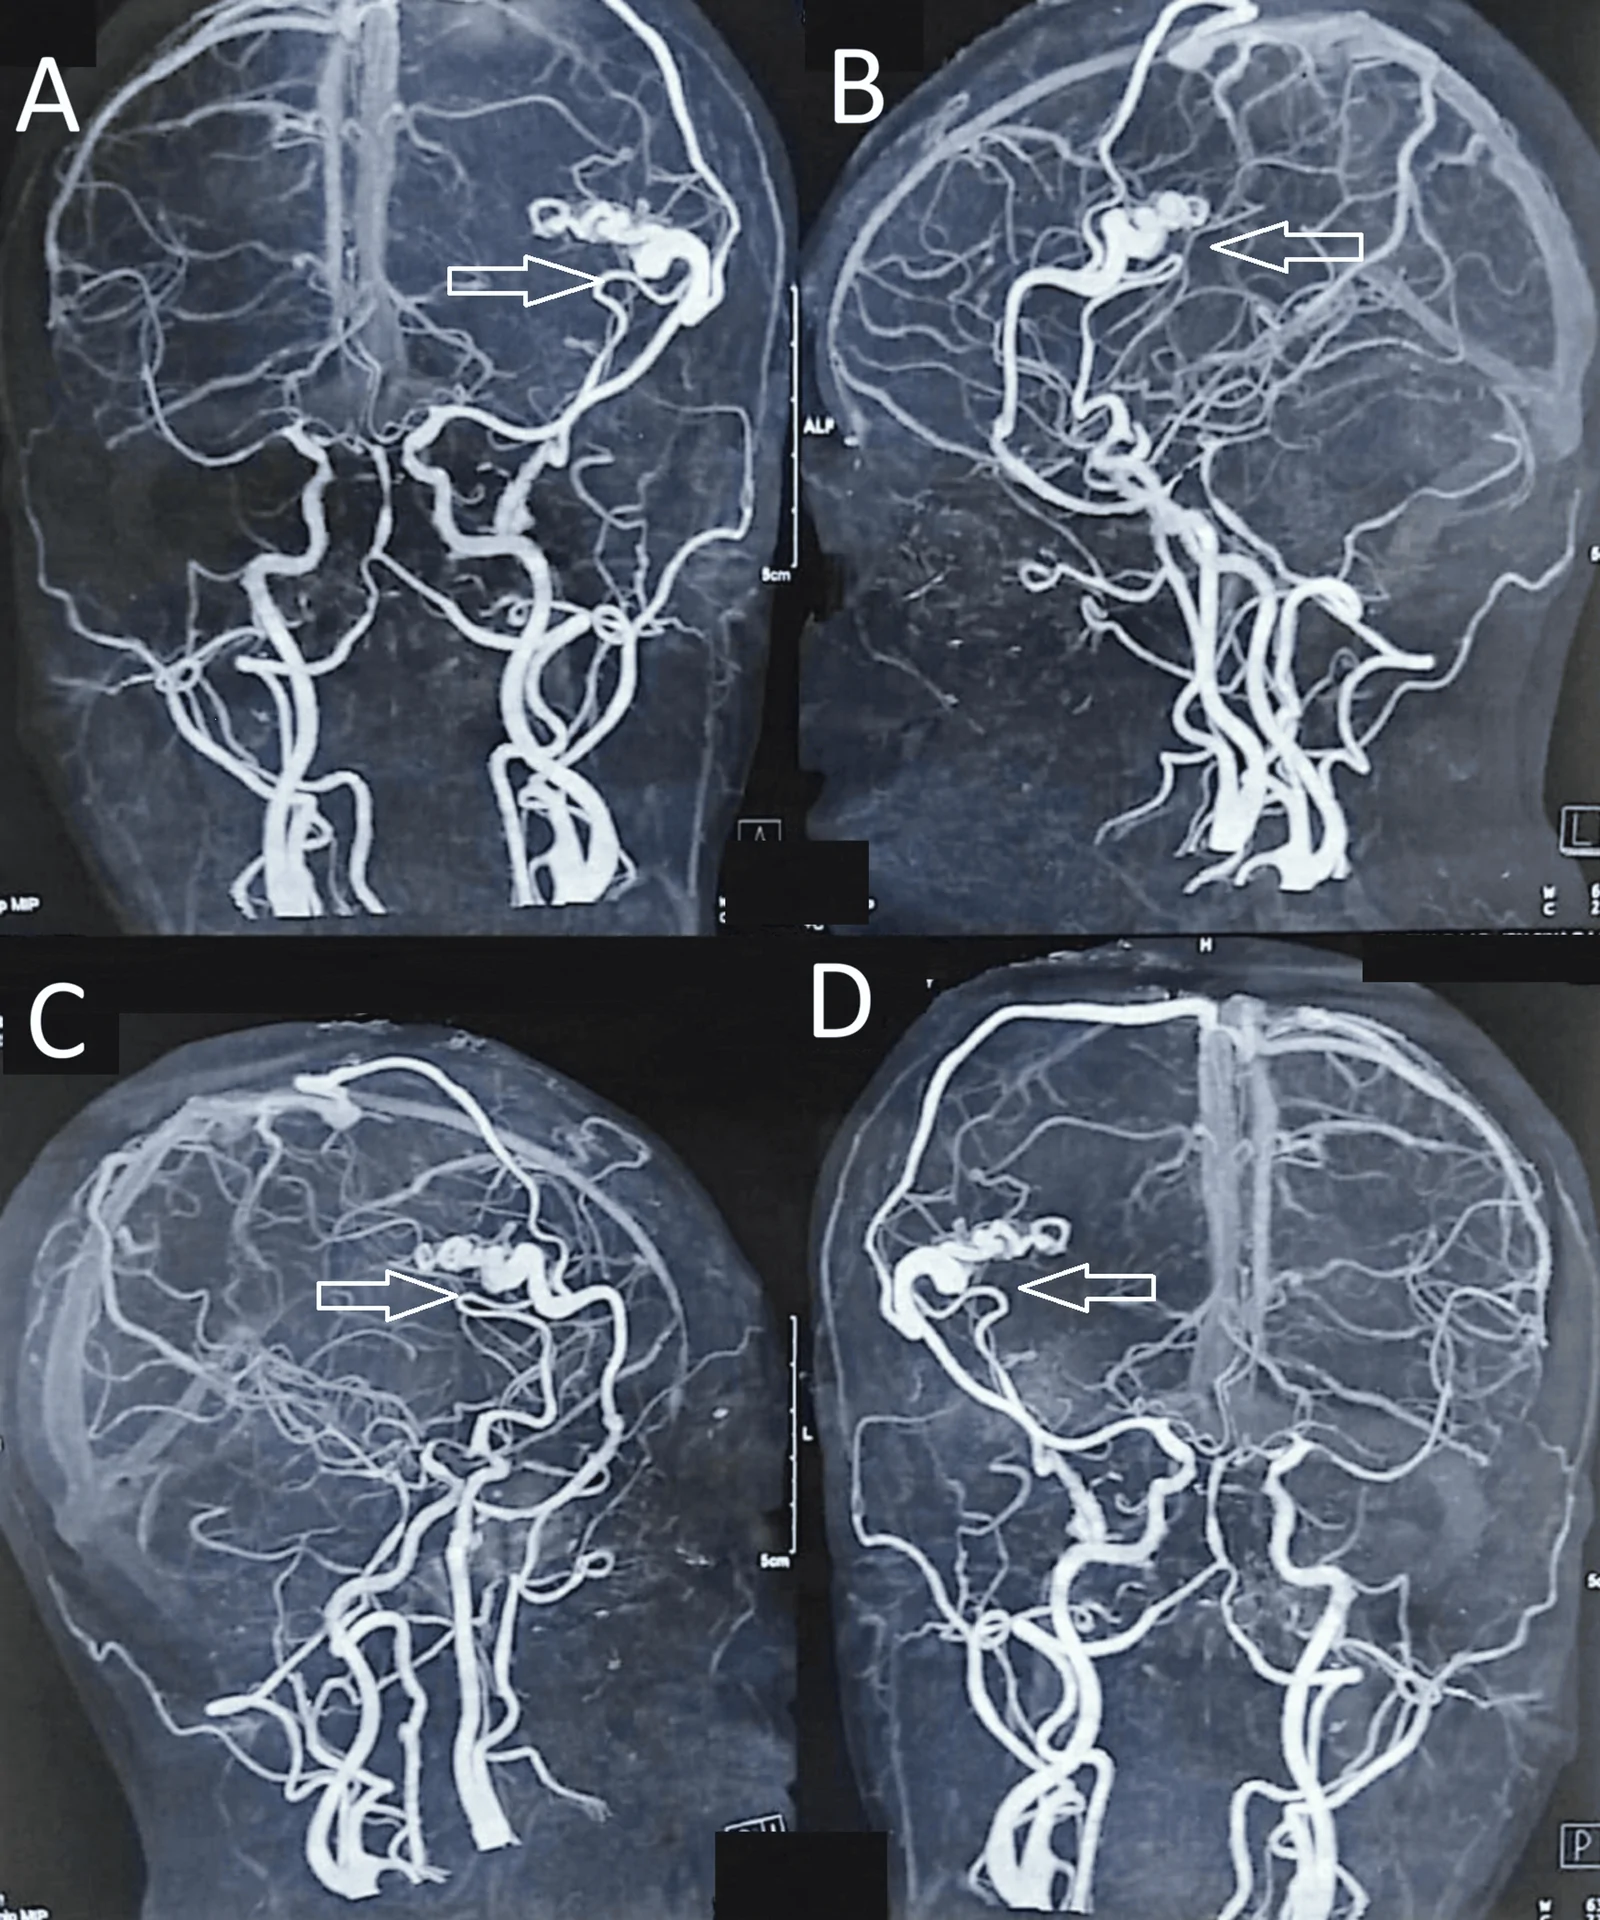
Computed tomography angiography demonstrating the cerebral AVM (A) Coronal view. (B) Sagittal view. (C) Oblique view. (D) Coronal venous reconstruction. White arrows indicate the AVM nidus. AVM, arteriovenous malformation
